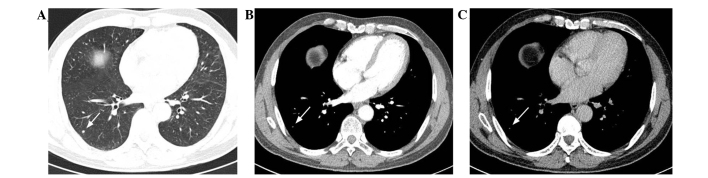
Plain chest computed tomography (CT) of a 58-year-old male with a pulmonary inflammatory myofibroblastic tumor. (A) Lung window setting revealing a small nodule with a maximum diameter of 5 mm located in the peripheral parenchyma of the right lower lobe. Due to its small size, contrast-enhanced CT images in (B) the arterial phase and (C) the venous phase were unable to reveal the degree of contrast enhancement of the nodule.
